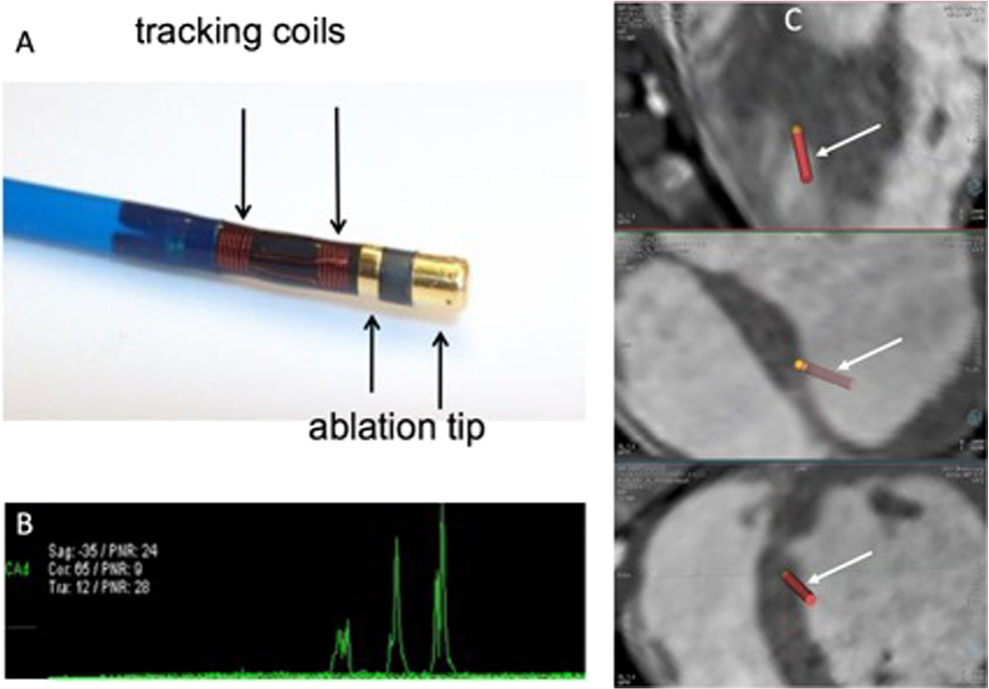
Active catheter tracking using a MR-compatible catheter with gold-tip electrodes and two 2.5-mm solenoid receive coils (a). Using a dedicated tracking sequence, the *X*, *Y* and *Z* coordinates of the catheter micro-coils can be determined in 3D space (b) whilst the catheter tip can be displayed as a red icon in three orthogonal MRI projections to guide a MR-EP procedure (c)
